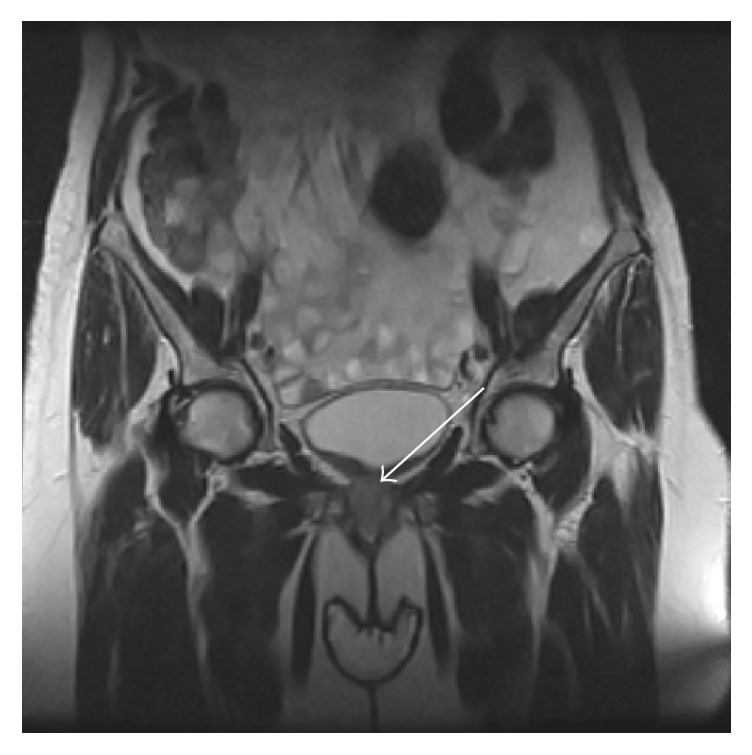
Coronal plane pelvic MRI showed an irregular mass with crenulated margins occupying the urethra and vagina.
